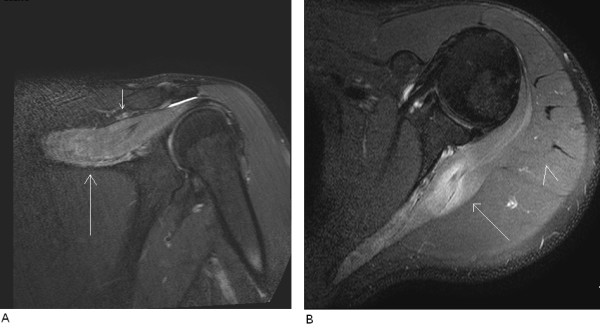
MRI examination performed three months following the initial symptoms. (A) Oblique coronal T2-weighted/turbo spin echo fat suppression image of the left shoulder. The long arrow indicates diffuse high signal intensity within the supraspinatus muscle, suggesting denervation changes. The short arrow indicates the suprascapular notch free of pathology. (B) Axial T2-weighted/turbo spin echo fat suppression image of the left shoulder. The long arrow indicates diffuse high signal intensity within the infraspinatus muscle, which suggests denervation changes. The arrowhead indicates the adjacent signal from the deltoid muscle, which appears normal.
